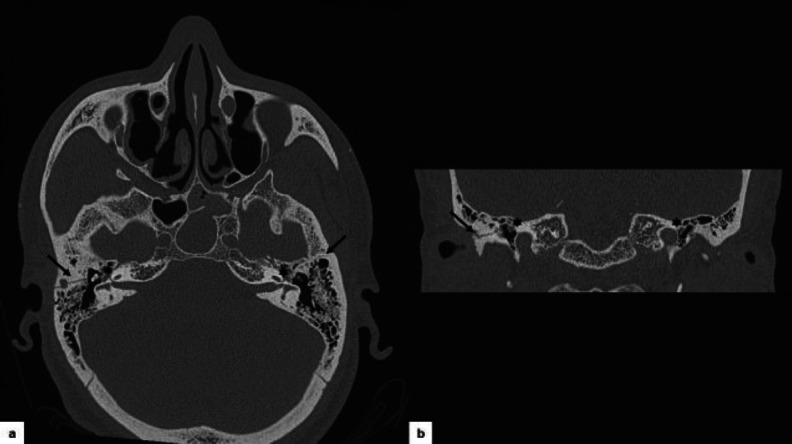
HRCT demonstrating a bilateral longitudinal fracture of the petrous bone (black arrow) with direction to the geniculate ganglion (asterisk). a. Axial section. b. Coronal section
